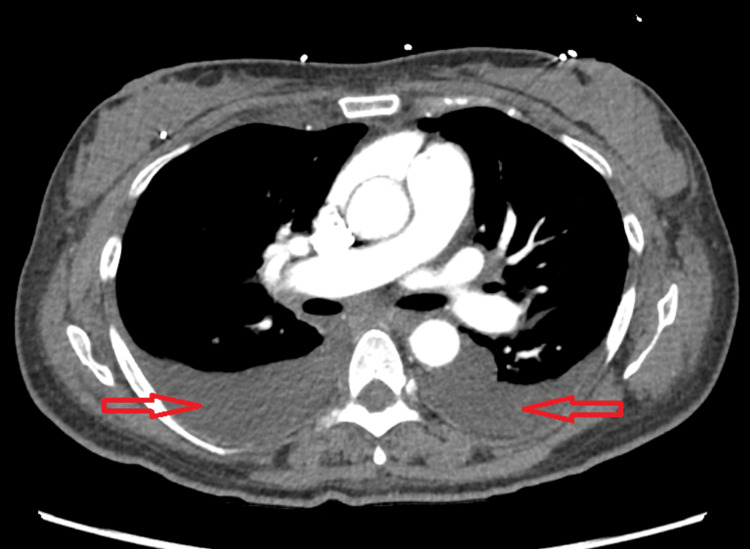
Axial view of computed tomography (CT) angiogram of the chest showing moderate bilateral pleural effusions on the day of admission (shown by red arrows).
